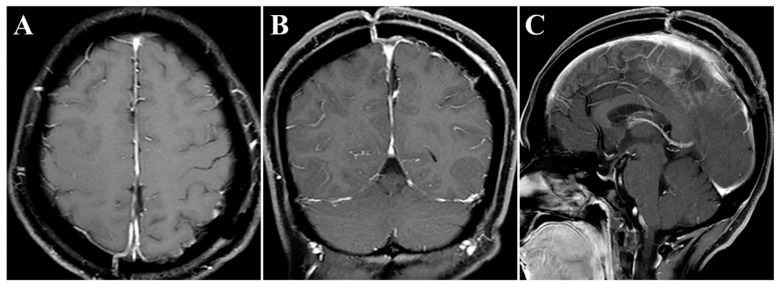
Postoperative contrast-enhanced MRI images. (A–C) Six months after surgery, MRI indicated regained parenchymal normal morphology. Axial (A), coronal (B), and sagittal (C) images.
